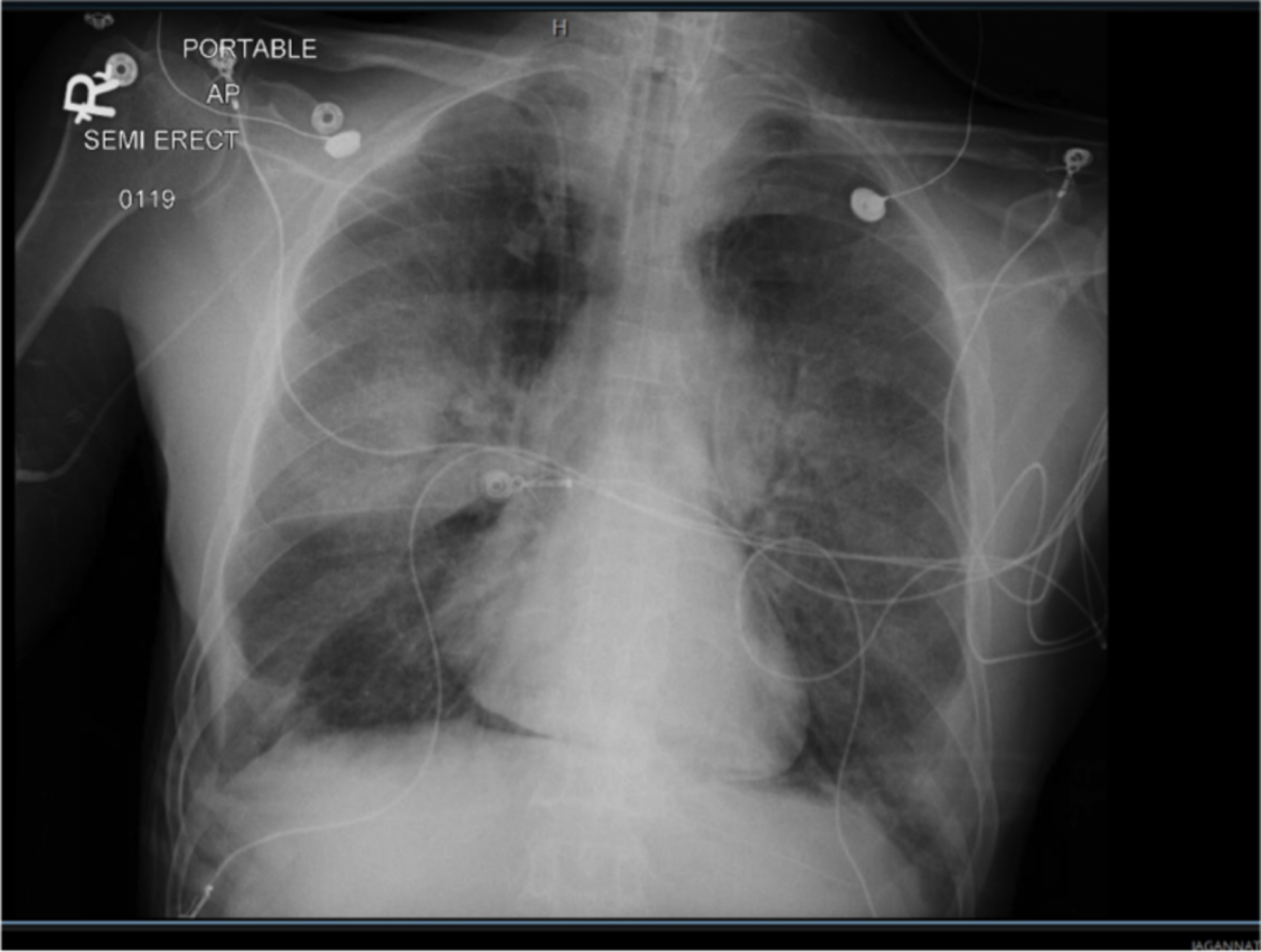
Chest X-ray (CXR) two days following TMP-SMX demonstrating worsening pneumomediastinum and infiltrates. TMP-SMX: Trimethoprim-sulfamethoxazole
